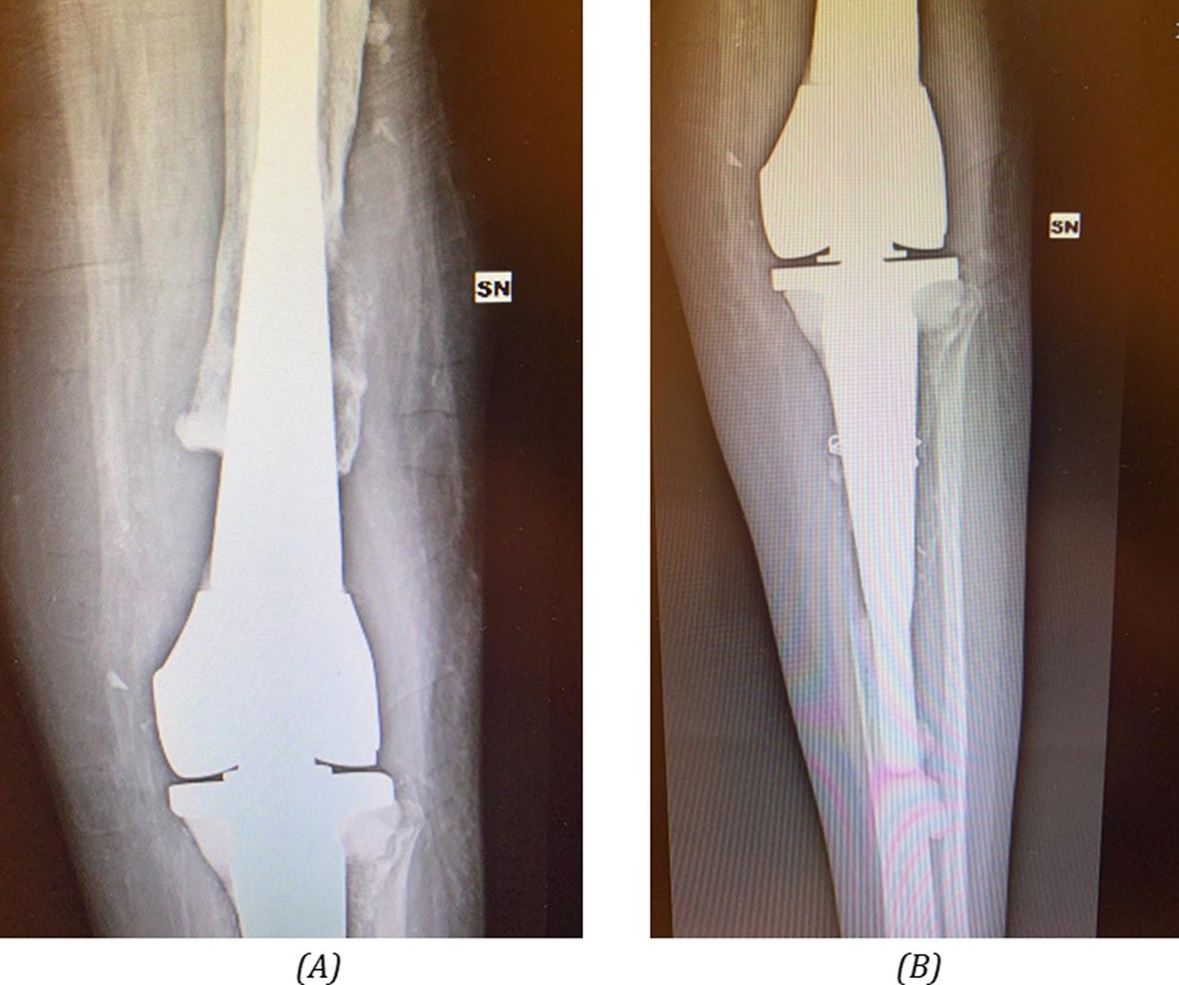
Post-implant X-rays of femoral (A) and tibial (B) custom-made cone
